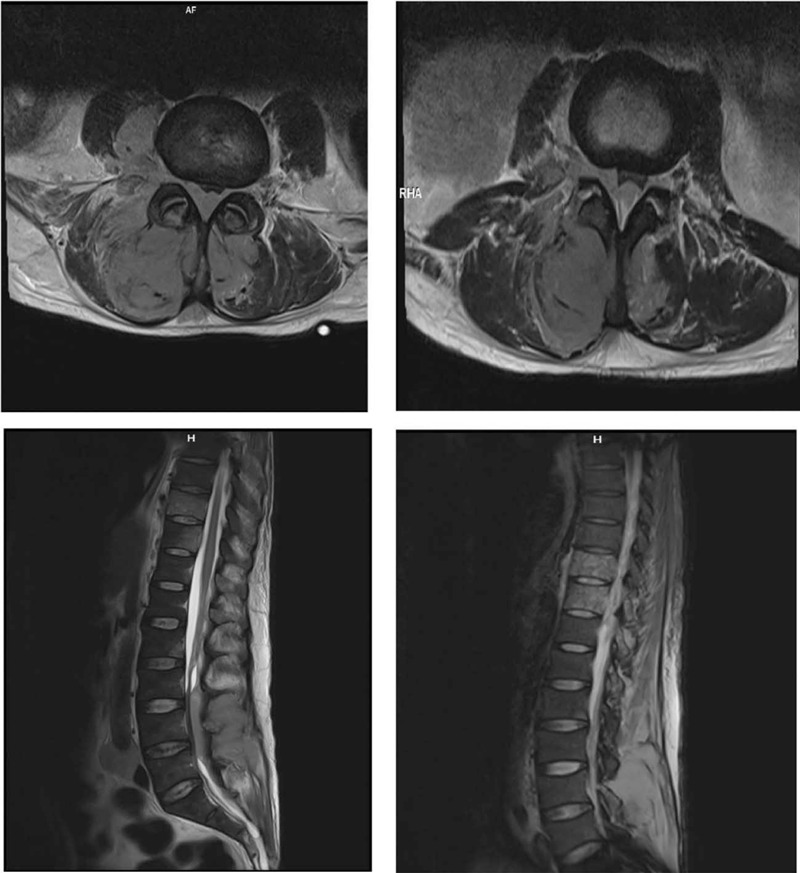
MRI sequence. MRI = magnetic resonance imaging.
